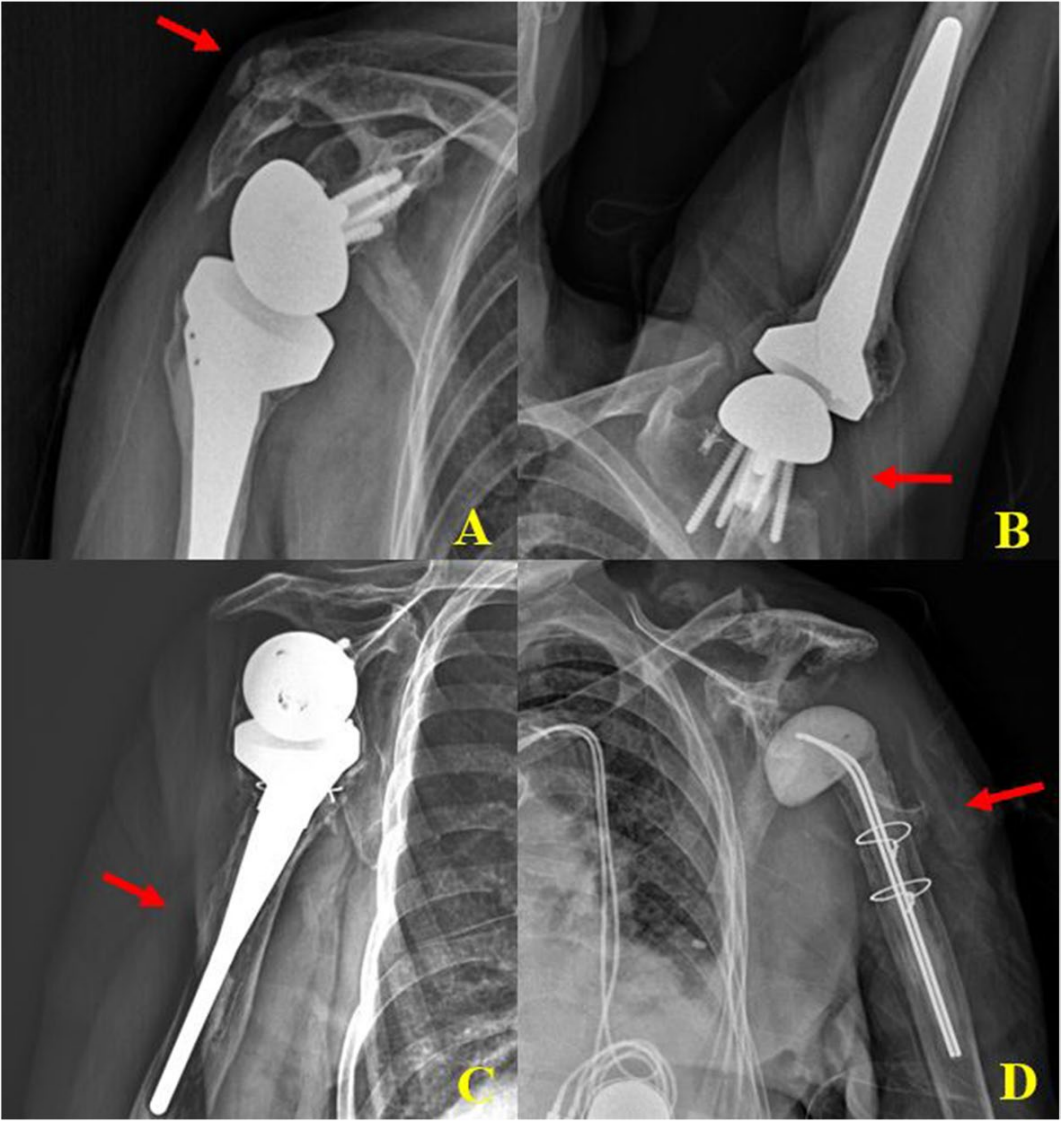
Radiologic complications of glenoid lateralization reverse total shoulder arthroplasty. Acromion fracture with scapular neck fracture (A). Axillary lateral view showing Grade 4 scapular notching (B). Humeral stem loosening was found in a 5-year follow-up X-ray (C). PROSTALAC insertion after implant removal was performed in a late infection (postoperative 3 years) (D)
